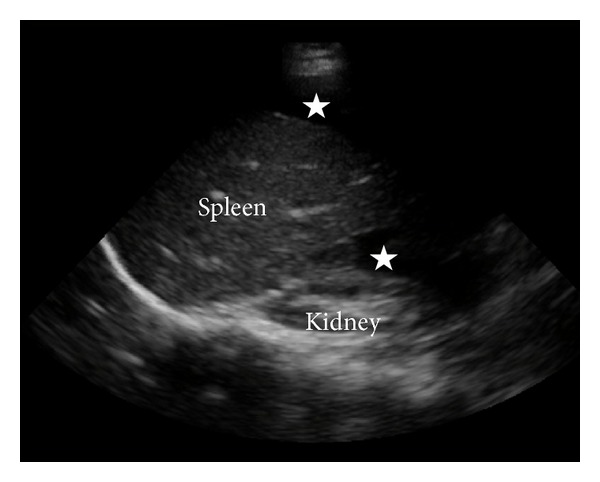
Left upper quadrant screenshot of bedside ultrasound performed in Case 2. The stars indicate surrounding anechoic blood.
